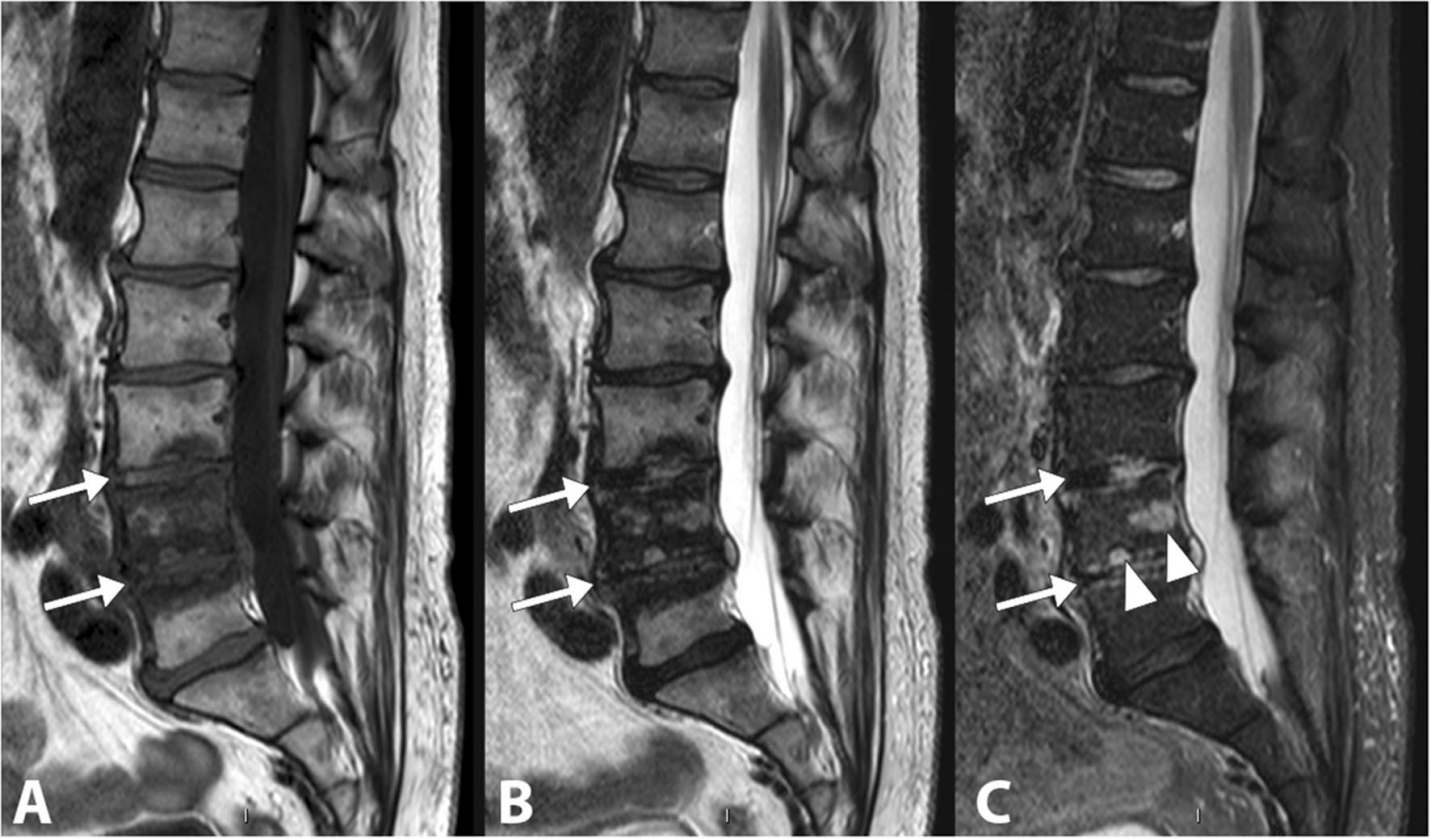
Gradually resolving lumbar (L3–L4–L5) spondylodiscitis caused by Candida  tropicalis. The sagittal T1-weighted (a), T2-weighted (b) and fat saturated T2-weighted (c) images show an altered signal intensity in correspondence with the L3–L4 and L4–L5 discs (arrows). Some infectious foci are still visible in the vertebral body of L4 (arrowheads)
